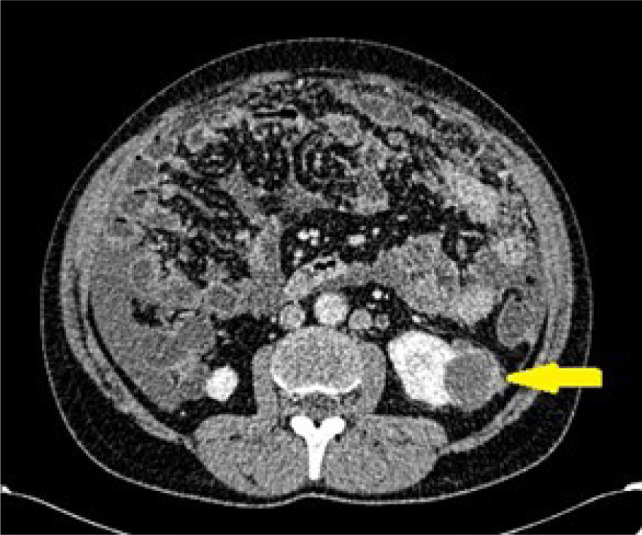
Axial CT at the level of the lower poles of the kidneys in a 43-year-old male showing a 37 mm bi-loculated left renal cyst (yellow arrow) with mild focal enhancing of its wall, categorized as Bosniak IIF cyst, confirmed by no change in size or morphology on 12 months follow-up. The angular interface sign is absent.
